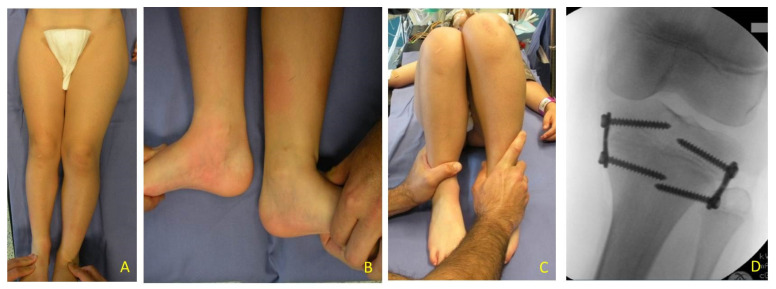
Same patient as shown in Figure 3, one year later: (A) LLD (leg length discrepancy) increased; (B) a severe LLD of 4 cm is present; (C) tibia shows major discrepancy; (D) postoperative X-ray showing performed PTE (temporary proximal tibia epiphysiodesis).
